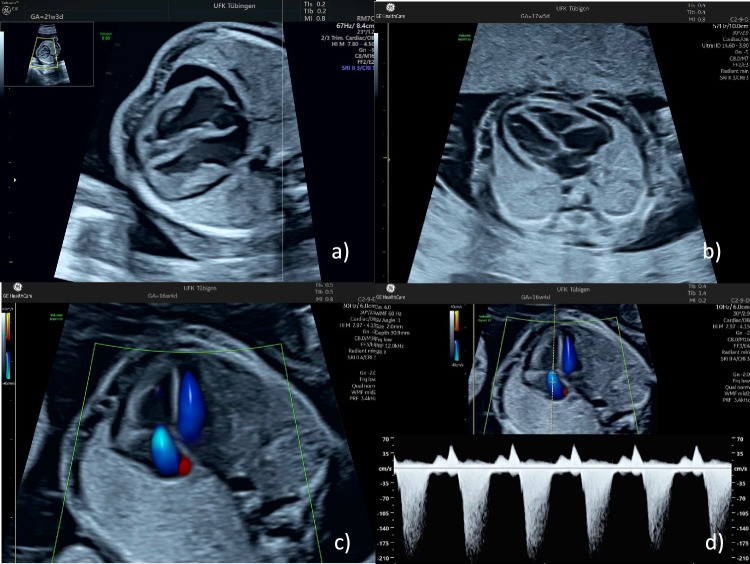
Typical fetal cardiac signs of a parvovirus B19 infection. The upper row of images shows a pericardial effusion (a) and cardiomegaly (b). The lower two images demonstrate tricuspid regurgitation by color Doppler (c) and spectral Doppler sonography (d)
